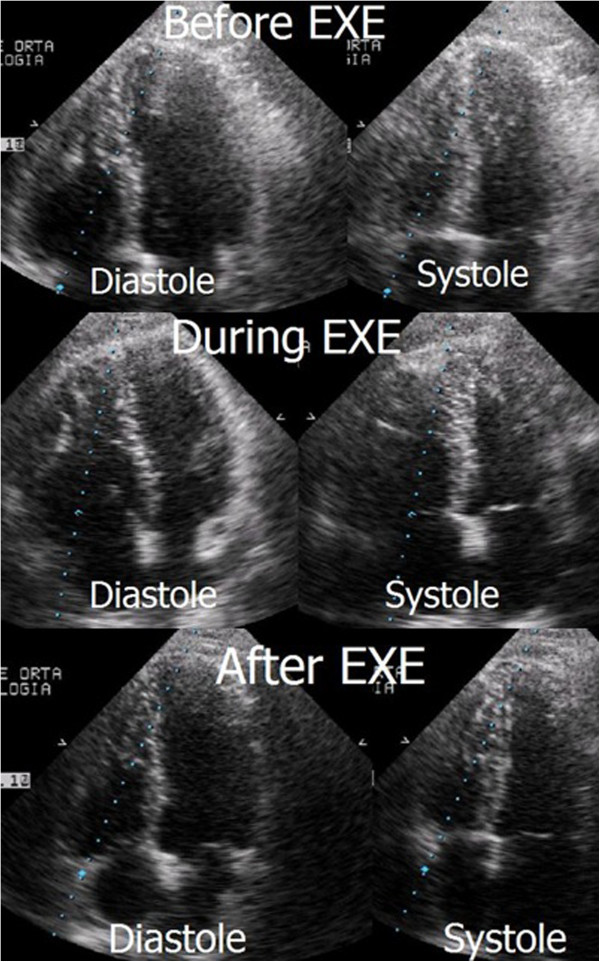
Apical four chamber view before, during, and after exercise with right ventricle dilatation only visible during exercise.
